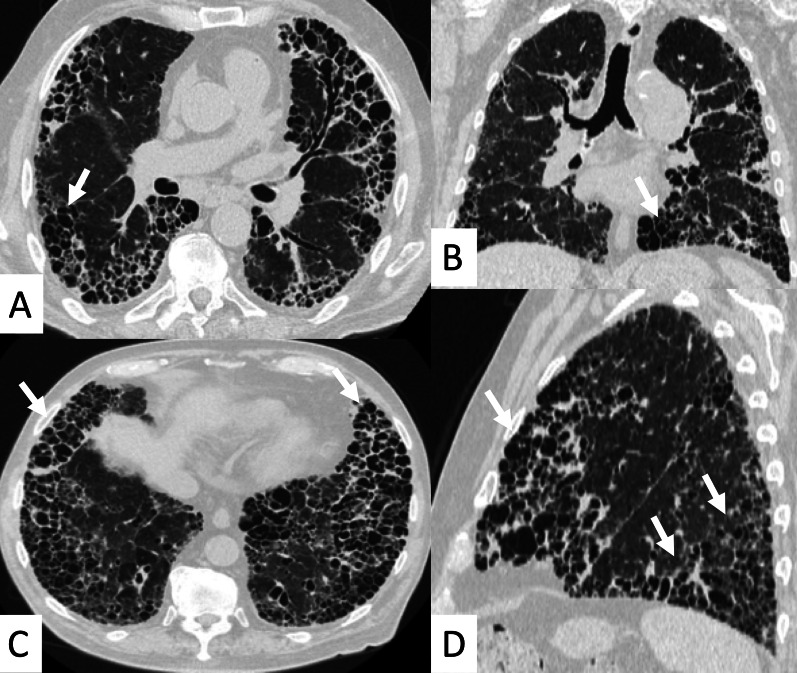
Axial (A, C), coronal (B) and sagittal (D) HRCT images demonstrate—in an RA patient—the presence of a florid honeycombing, involving more than 50% of the lung. This finding is typically due to macrocystic spaces (white arrows) and has been defined as “exuberant honeycombing sign”
